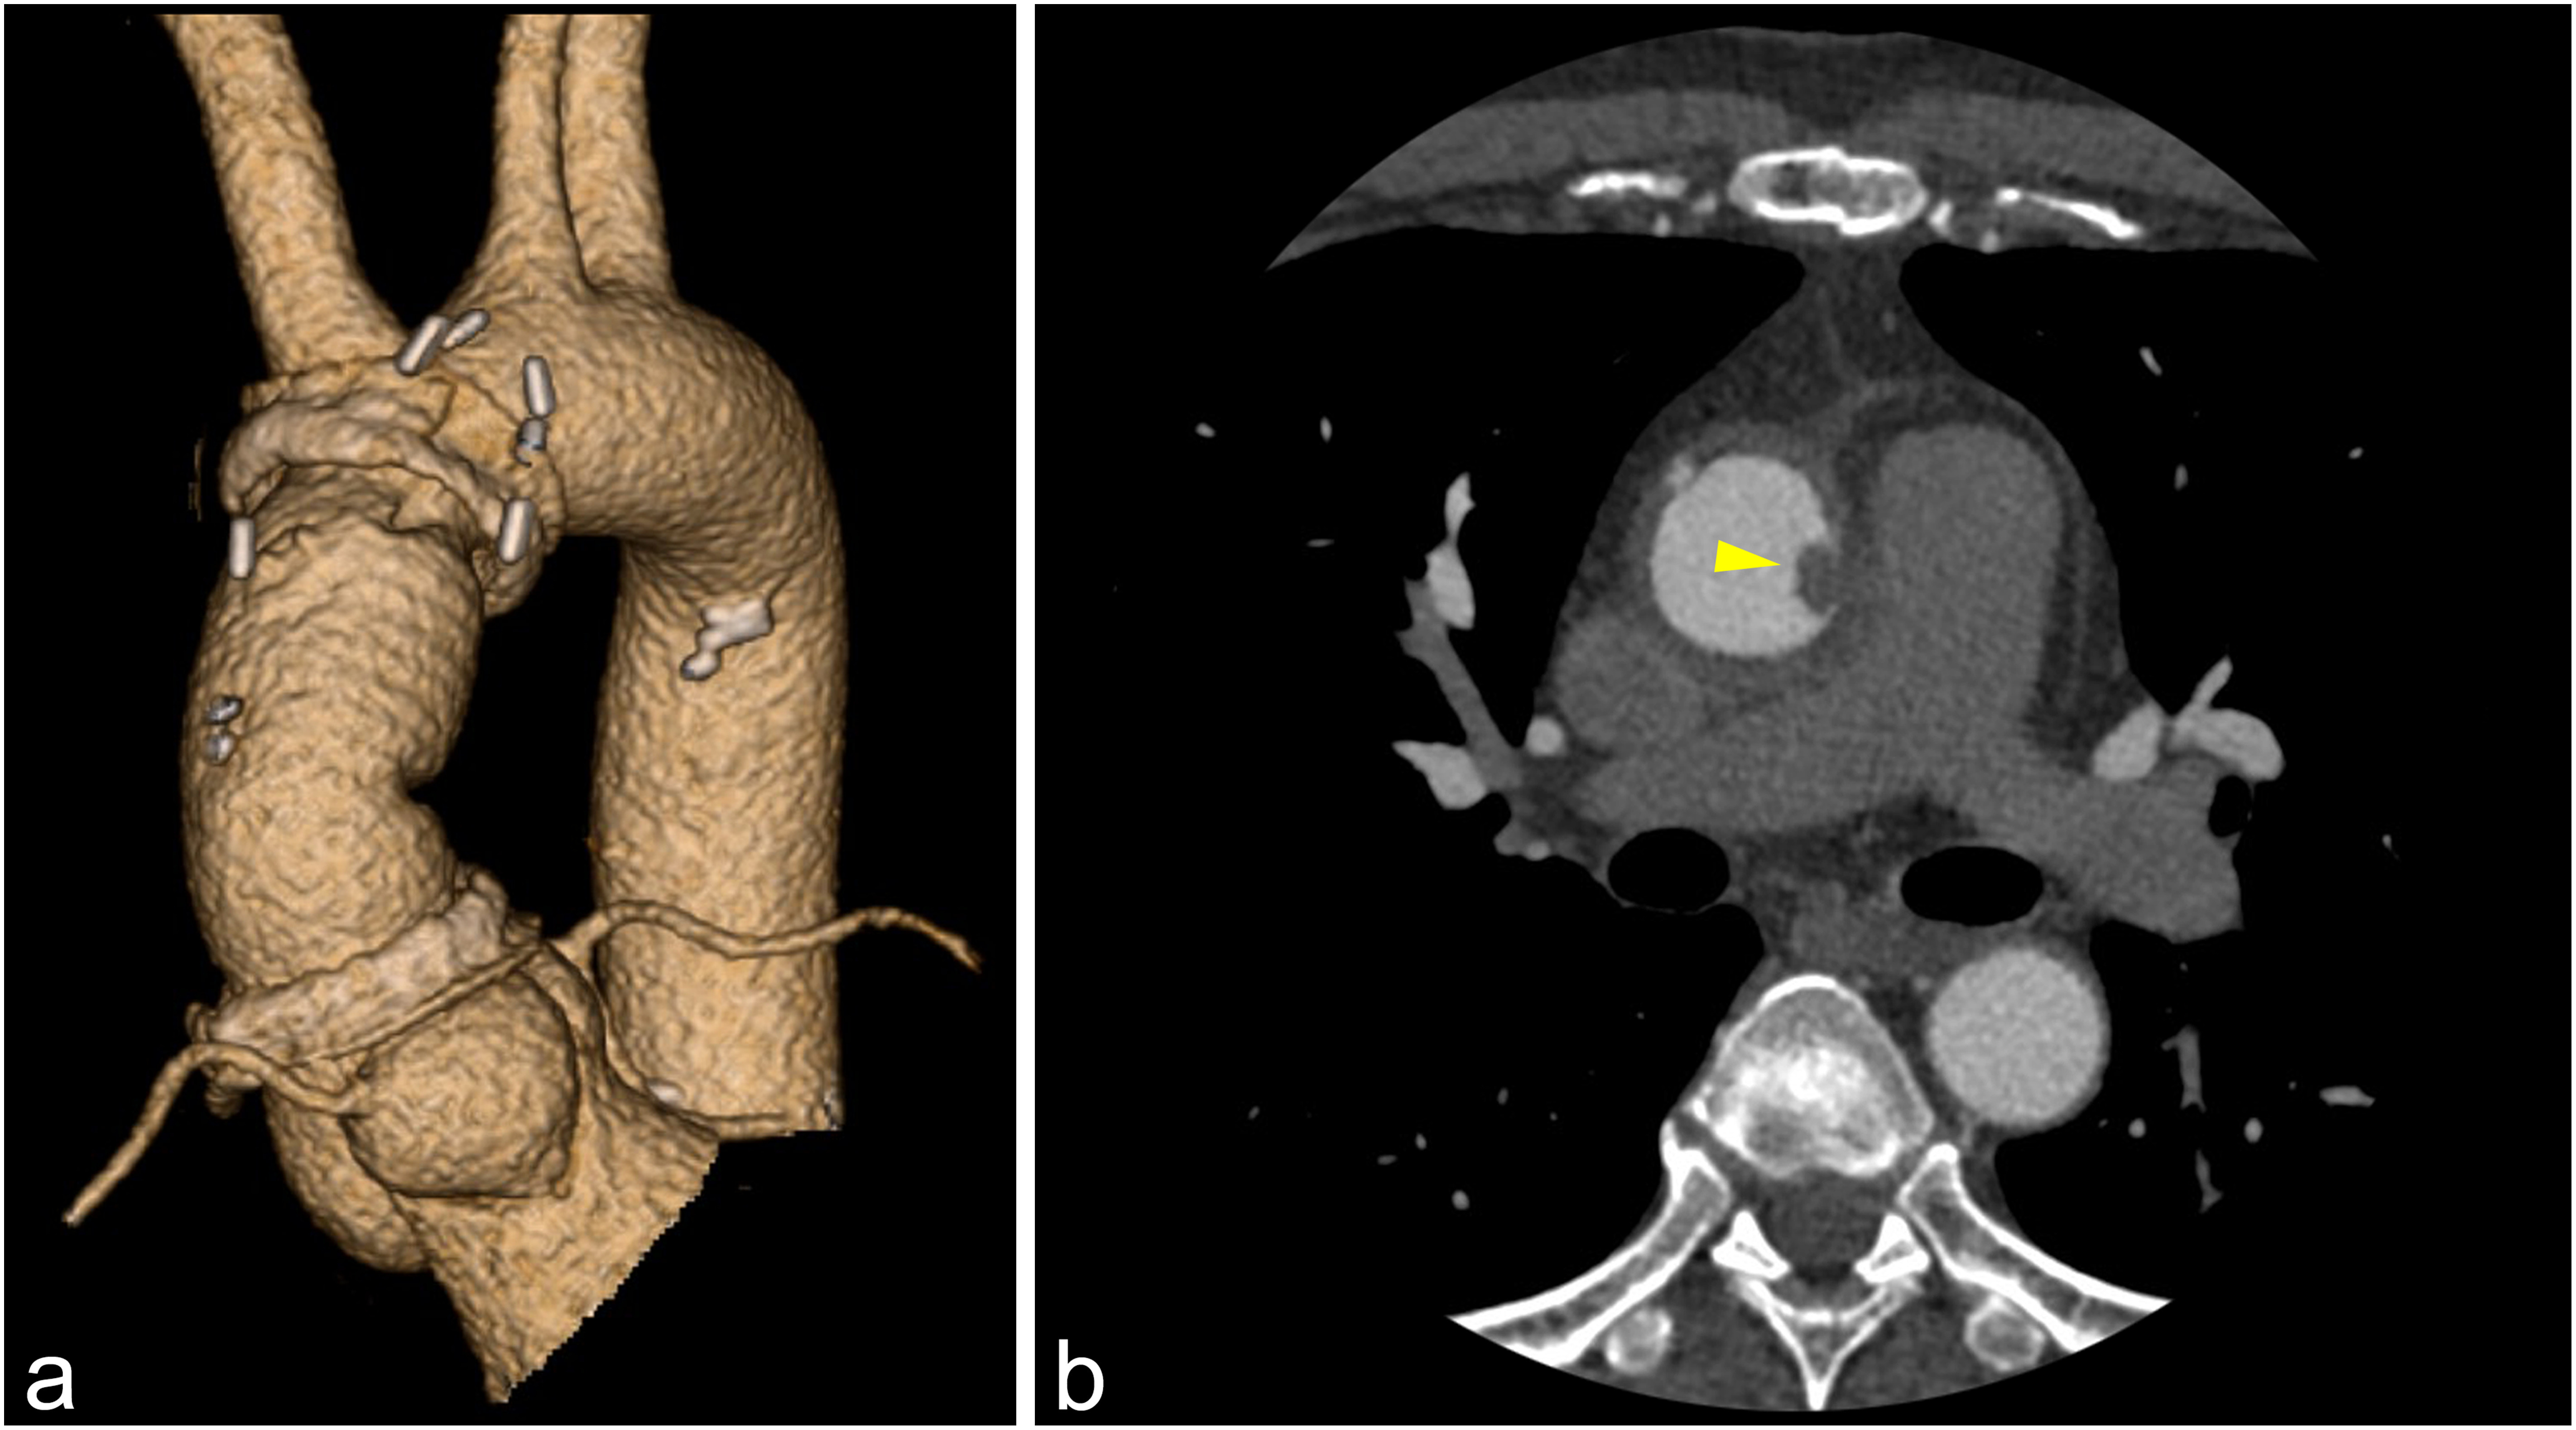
Fig. 3 Postoperative computed tomography scans at (a) 1 week and (b) 6 months after surgery. The arrowhead indicates a thrombus formed inside the graft.
